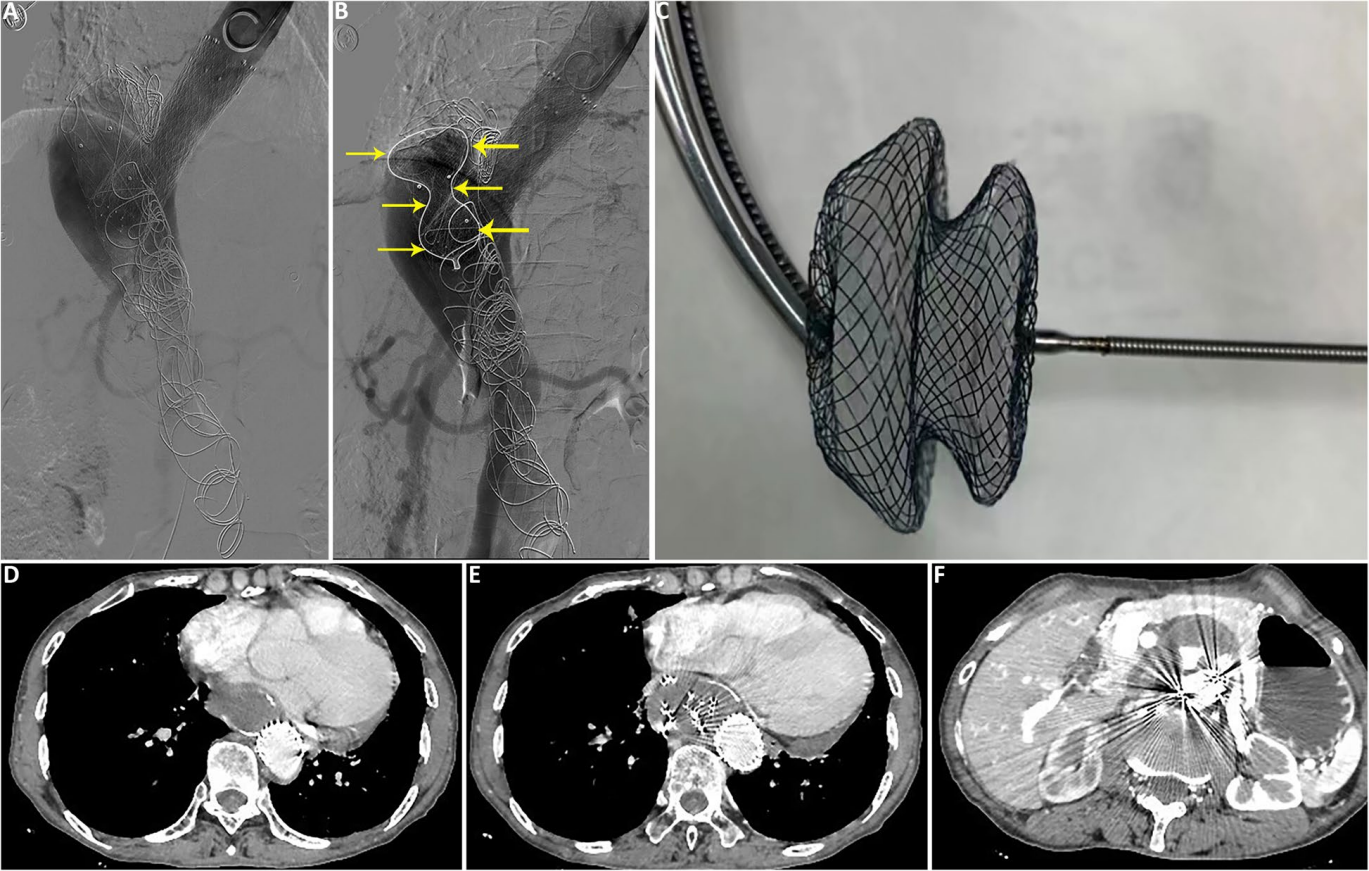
Atrial septal defect occluder combined with covered stents to embolize the false lumen of post-aortic dissection aneurysm. A The covered stent was implanted into the false lumen of the aortic dissection through the distal rupture. B An atrial septal defect occluder was inserted into the covered stent; C Image of an atrial septal defect occluder. D-F Six months after the procedure, CTA showed obvious thrombus in the false lumen
